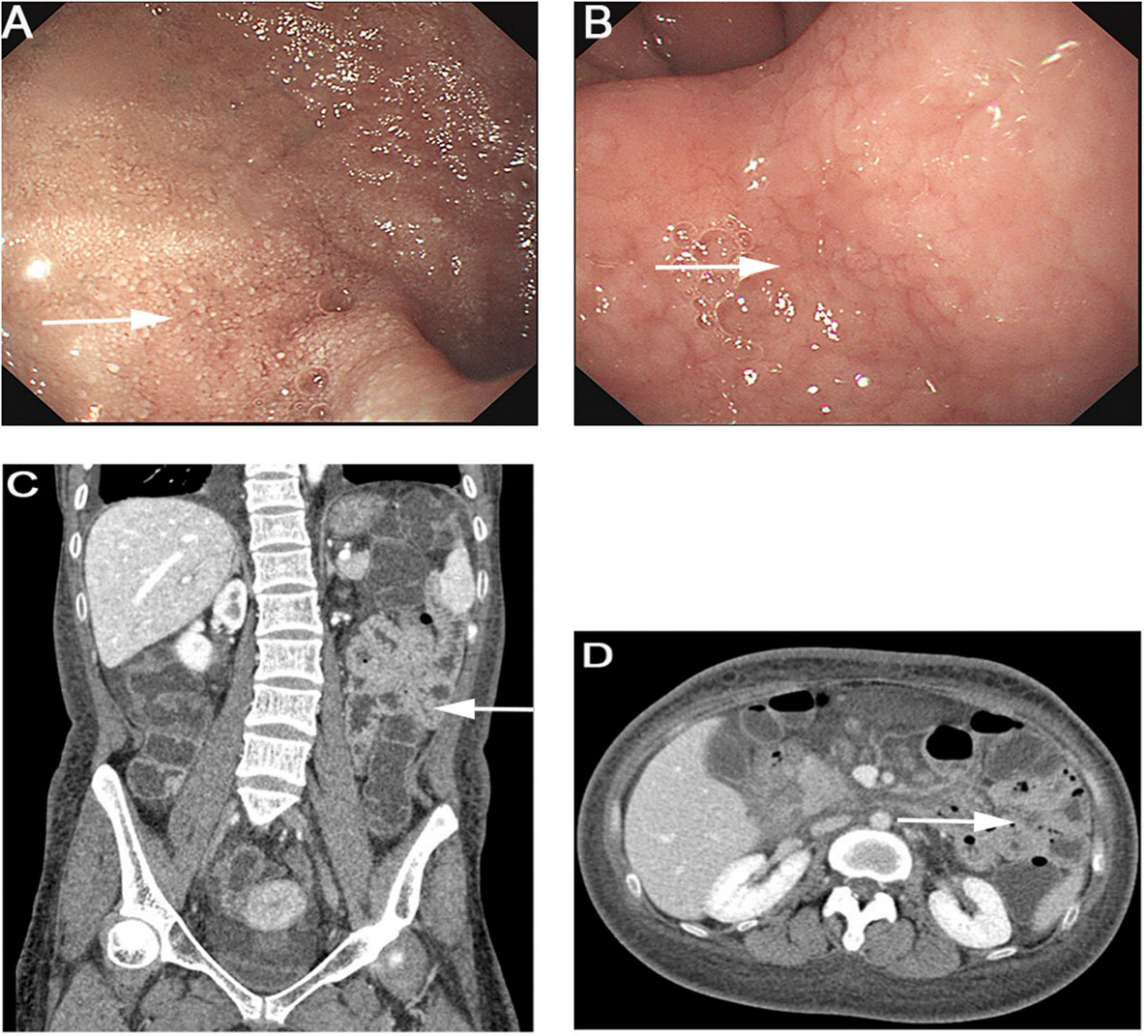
a shows the snowflake appearance of the duodenum and b shows the granuloid changes in the gastric antral mucosa by electronic gastroscopy. Reconstructive CT of the small intestine in (c & d) demonstrate segmental thickening of the intestinal wall with local intestinal stenosis. Arrows highlight the indicated features
